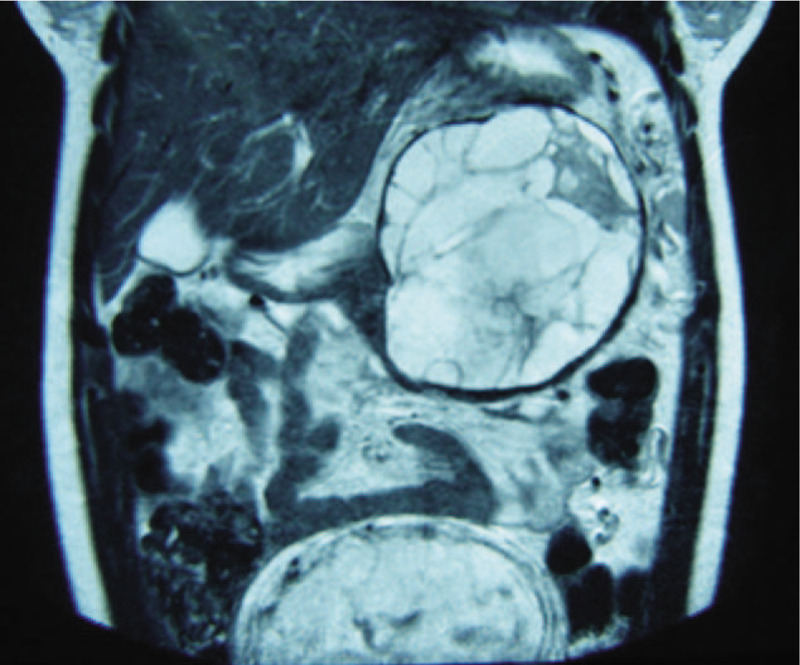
Magnetic resonance imaging showing an 11.8 cm × 11.6 cm × 9.5 cm multi-lobed neoplasm, arising from the body and tail of the pancreas with thin internal septations.
